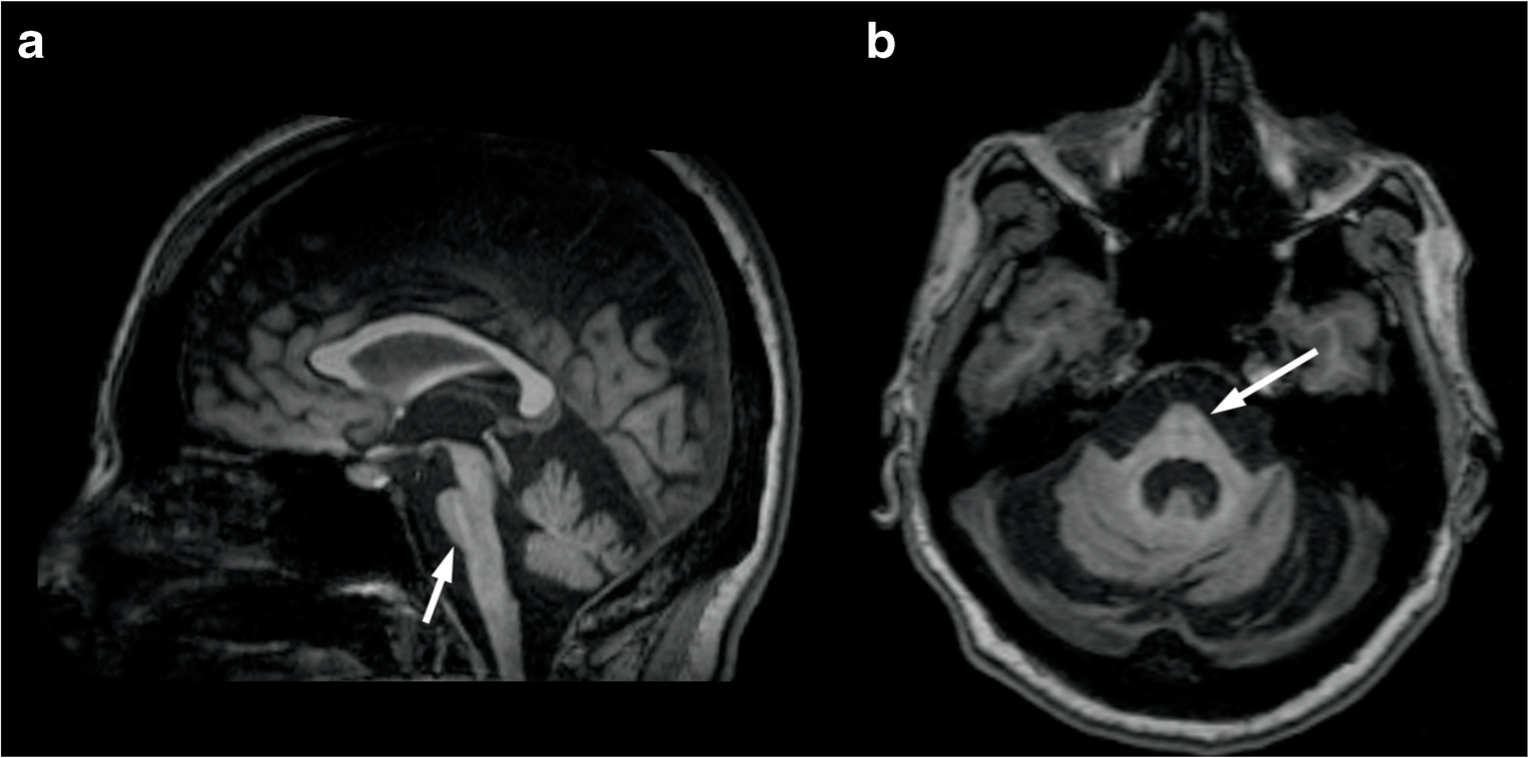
Imaging findings in a 38-year-old female SCA2 patient. Sagittal (a) and axial (b) multiplanar reconstructions of 3D T1-weighted volume show a global cerebellar volume loss, involving both the vermis and cerebellar hemispheres, along with significant brainstem atrophy, particularly affecting the pons which demonstrates a flattening of its inferior profile (arrows)
